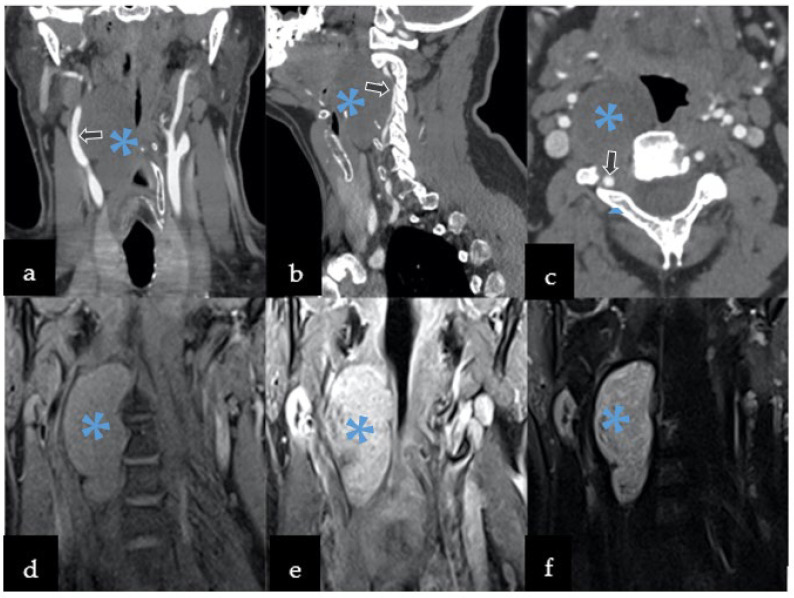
Preoperative imaging included both computed tomography angiography (CTA) and magnetic resonance imaging (MRI). CTA revealed hypodense well-defined mass with calcification displacing the vessels in the following planes: (a) coronal, (b) sagittal, and (c) axial. MRI was characterized by: (d) by low T1 signal, with (e) T1 contrast enhancement, and (f) heterogeneously hyperintense T2 signal. Legend: arrows—compression and displacement of the right carotid artery, *—lesion.
